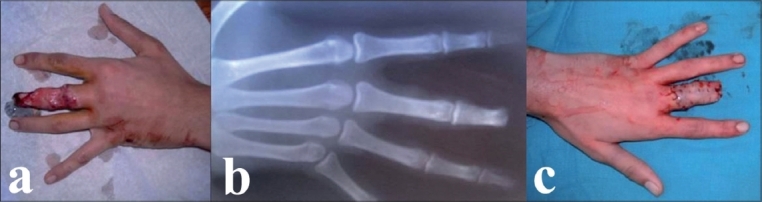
(a) Urbaniak Class III avulsion at the level of the DIP joint of left middle ray proximal phalanx. (b) Anteroposterior (AP) X-ray of left hand showing the level of amputation. (c) Postoperative appearance after skin graft
